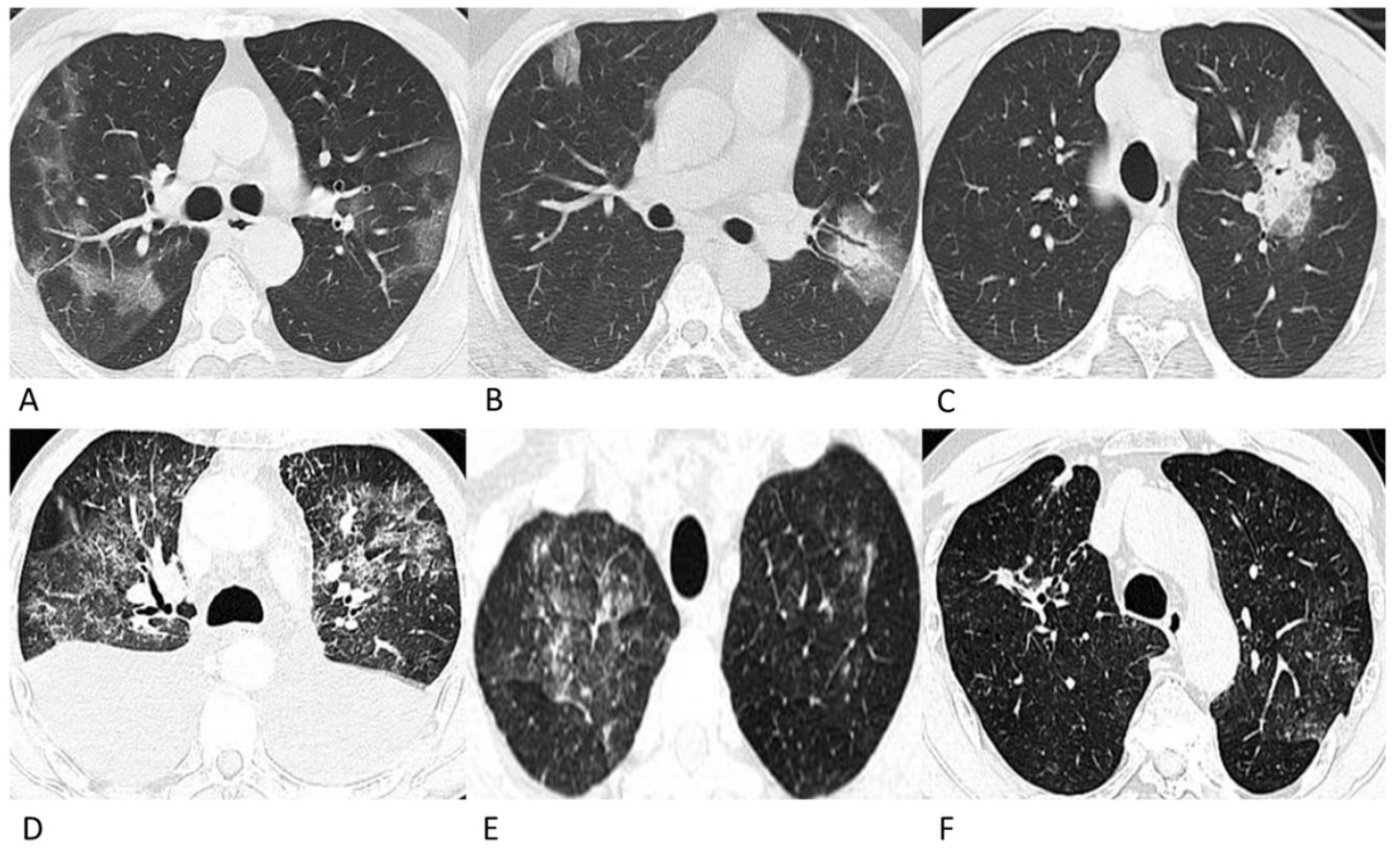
Representative chest CT images of COVID-19 pneumonia (A-C) and lymphoma-associated pneumonia (D-F). (A) A 62-year-old man who worked in Wuhan had a fever and chest tightness. Patchy GGOs are shown in more than one lobe. (B) A 42-year-old man, who was in close contact with a confirmed patient developed fever and cough on admission. Patchy GGOs in the peripheral right lung and mixed GGOs in the left lung are observed. Bronchograms are shown in the lesions. (C) A 28-year-old man who was in close contact with the confirmed patient in Fig. 1A developed fever. A single mixed GGO and consolidation is shown in the left lung. Focal interlobular septal thickening could be observed. (D) A 61-year-old man was diagnosed with ALK (+) anaplastic large cell lymphoma and received chemotherapy with CHOP before chest CT, and he did not have a fever or a positive epidemiological history. Diffuse patchy GGOs are shown in more than one lobe. (E) A 49-year-old man was diagnosed with diffuse large B-cell lymphoma, and received chemotherapy with rituximab before the chest CT. He had a fever during chemotherapy but had no epidemiological history of COVID-19. Diffuse patchy GGOs with several solid nodules are shown in both upper lobes. (F) A 69-year-old man was diagnosed with diffuse large B-cell lymphoma, and received chemotherapy with rituximab before the chest CT, and he did not have a fever or a positive epidemiological history. Slightly diffuse patchy GGOs are shown in the left upper lobe, and patchy consolidation was observed in the right upper lobe.
